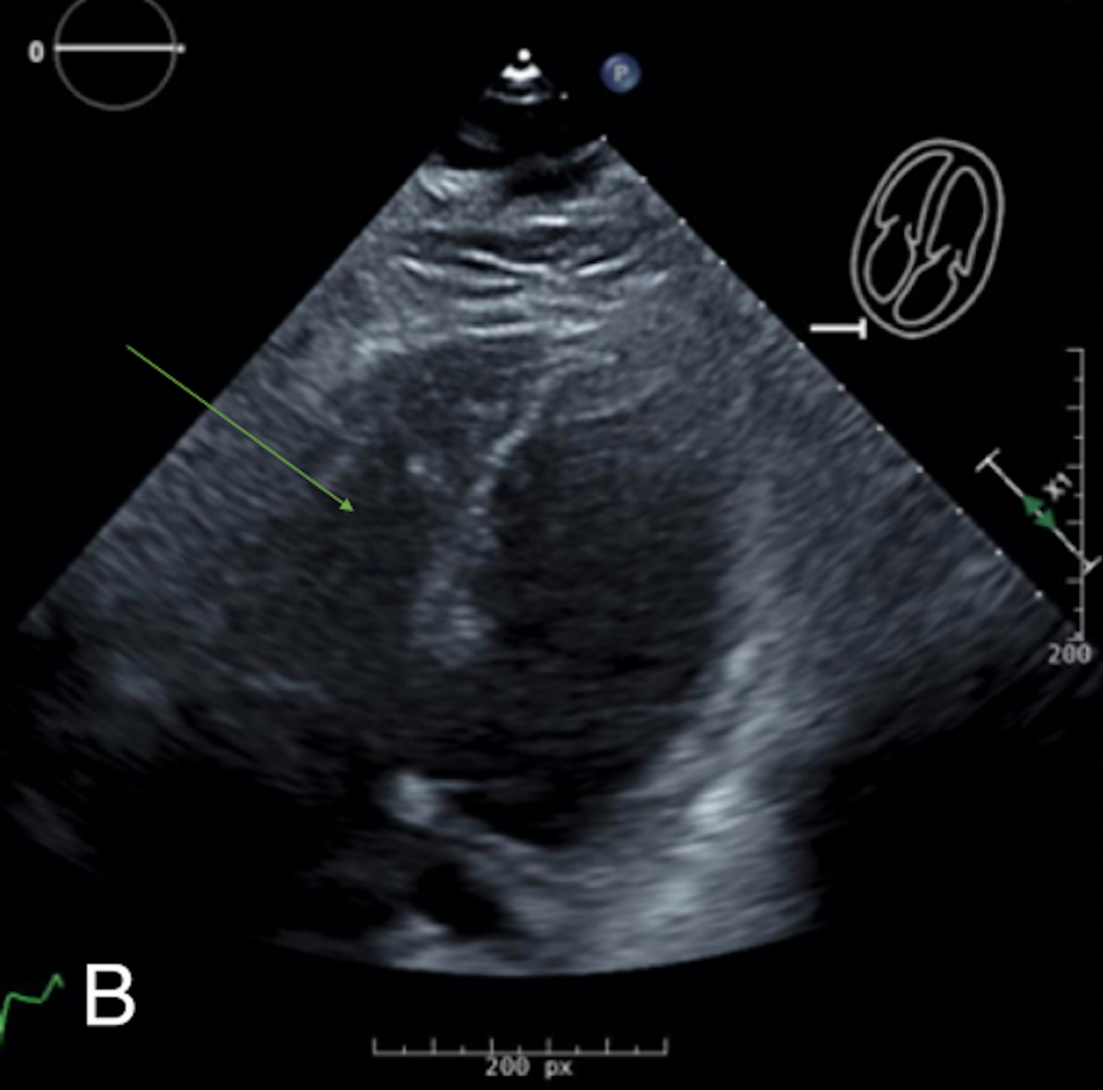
Repeat transthoracic echocardiogram one day after catheter-directed therapy shows drastic improvement in the size of the right ventricle (green arrow).
